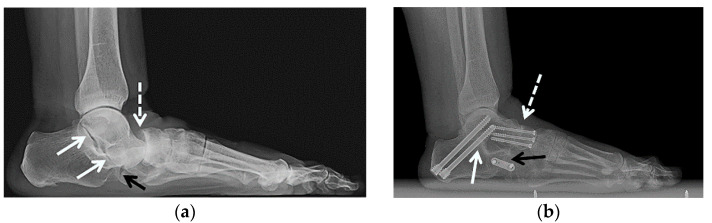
(a,b) A 64-year-old female with a history of traumatic right talonavicular dislocation several months prior presented with continued pain, pes planus with plantar valgus and equinus contracture and posttraumatic osteoarthritis, subsequently treated with triple arthrodesis. (a) Lateral weight-bearing radiograph of the right foot shows per planus with dorsal subluxation of the talonavicular joint and superimposed advanced osteoarthritis (dashed white arrow). Note the associated mild asymmetric narrowing of the subtalar (white arrows) and calcaneocuboid joints (black arrow). (b) Postoperative weight-bearing lateral radiograph shows interval triple arthrodesis with two Herbert screws transfixing the posterior subtalar joint (white arrow), three partially threaded cannulated screws transfixing the talonavicular joint (dashed white arrow) and a short plate with two screws transfixing the calcaneocuboid joint (black arrow) with solid bony bridging across the arthrodesis sites.
